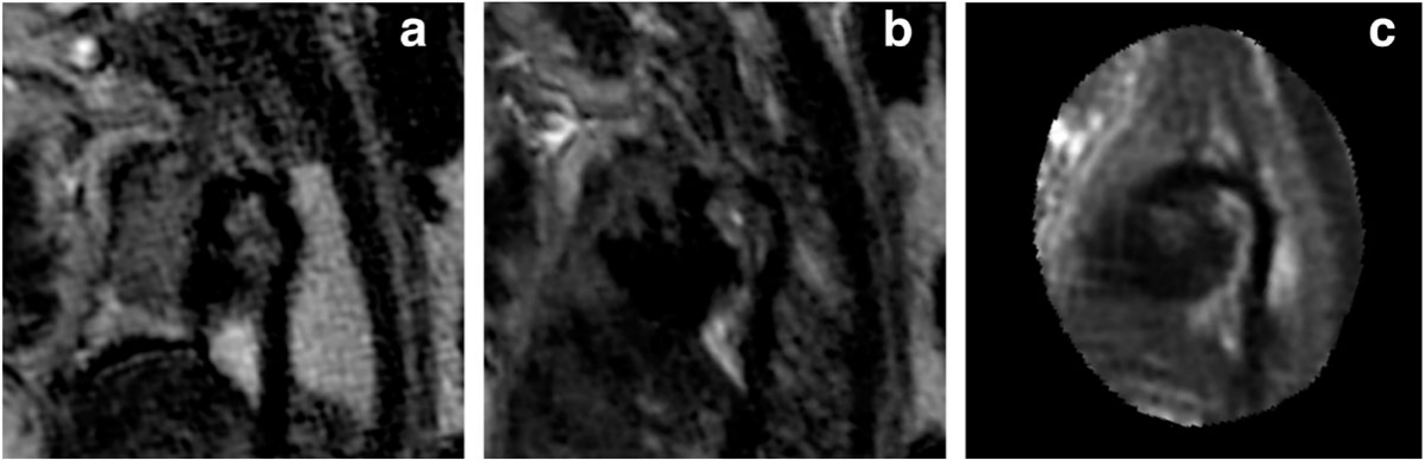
The aortic and ductal arches in a 36 weeks fetus as visualised from a.) a single-shot fast spin echo MRI image; b.) a (motion-corrupted) 3D volume derived from the concomitant multi-slice stack; and c.) the resultant 3D volume after application of a GPU accelerated super-resolution algorithm for slice-volume registration (using multiple stacks). In the final image, the hypoplastic aortic arch is now clearly visualised superior to the dominant ductal arch.
